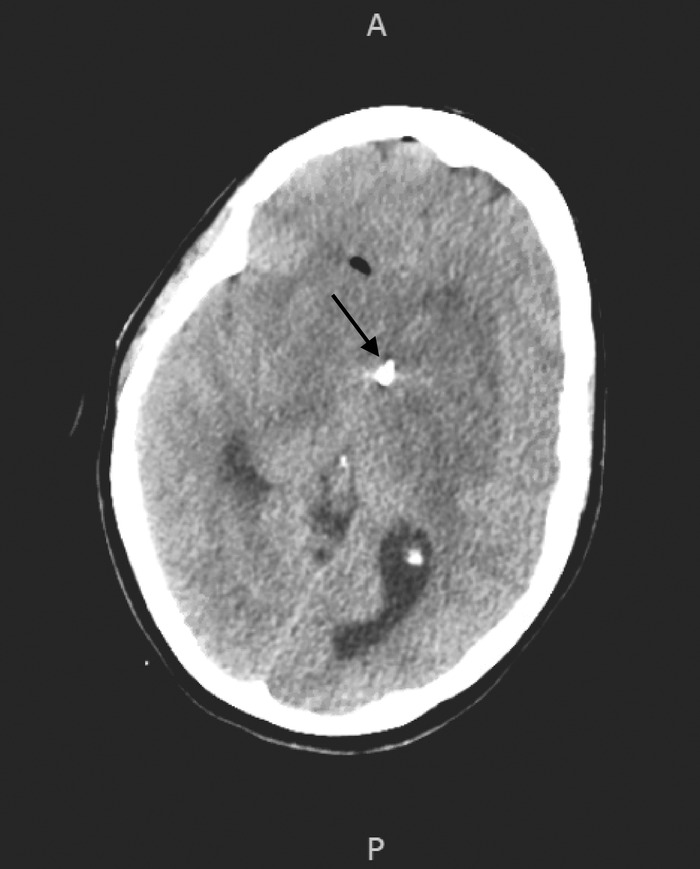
Computed tomography head after external ventricular drain placement (arrow).
